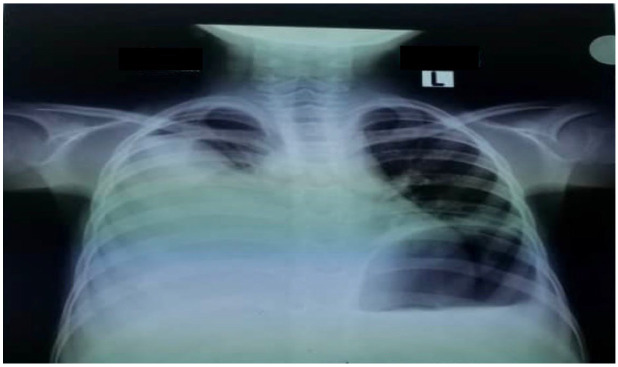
Chest X-ray of the patient showing right pleural effusion.
